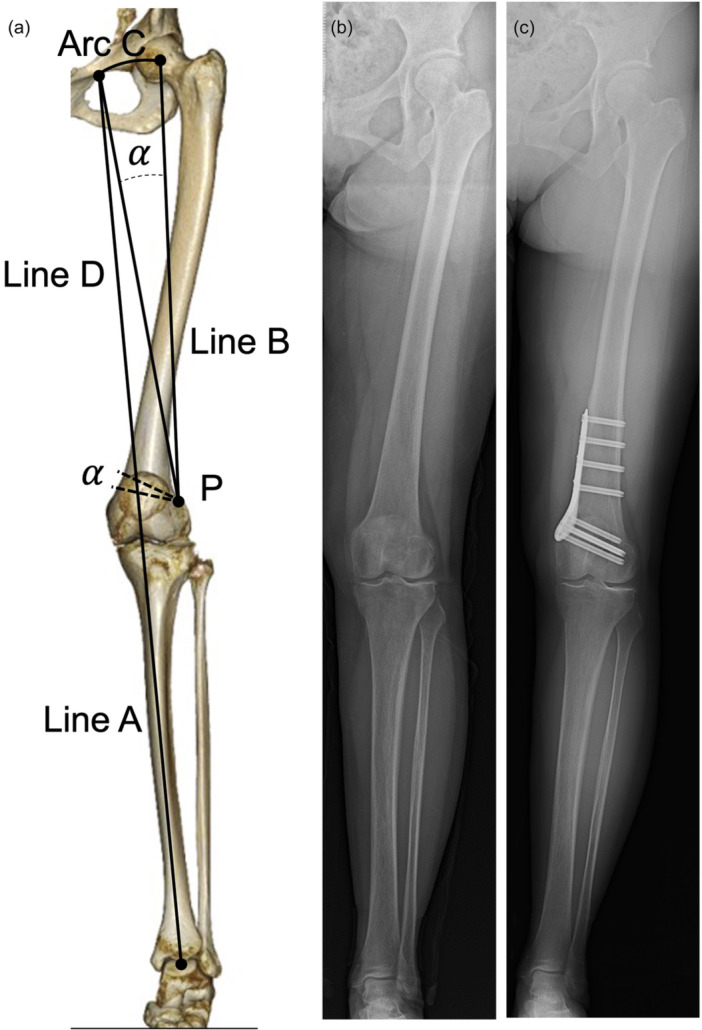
Preoperative planning and pre‐ and postoperative standing (full‐length lower‐limb anteroposterior radiographs for MCW‐DFVO). (a) Preoperative planning. (b) Preoperative standing. (c) Postoperative standing. MCW‐DFVO, medial closing wedge‐distal femoral varus osteotomy.
